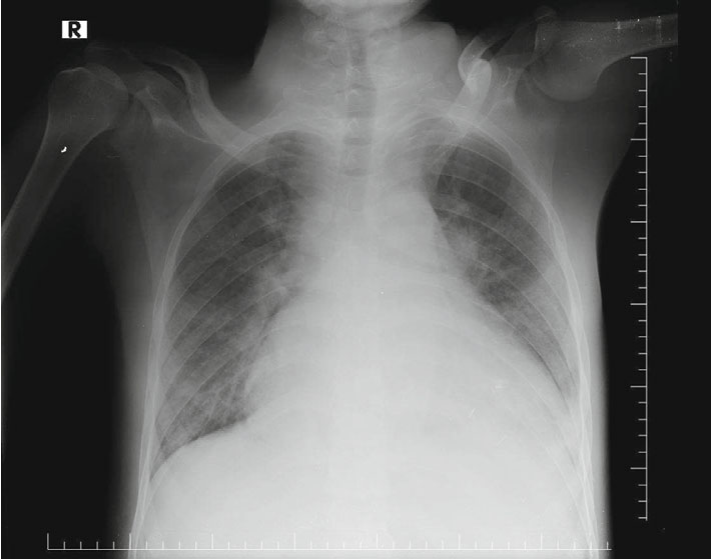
A chest X-ray demonstrated bilateral pleural effusion and cardiomegaly.
